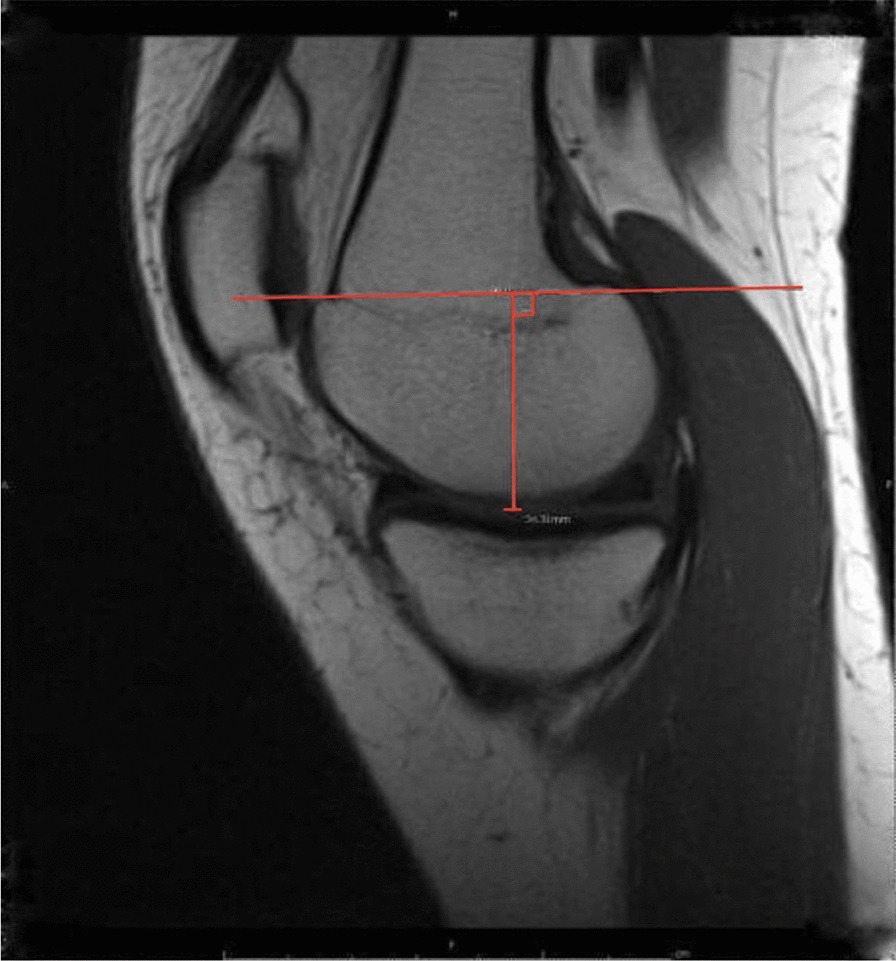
The measurement of medial femoral condyle sphere diameter on a T1 sagittal magnetic resonance images
